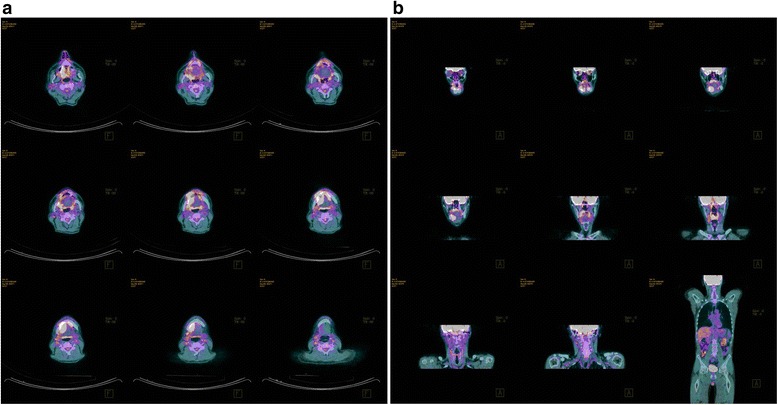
Preoperative ^18^F-FDG PET/CT. 2a axial plane; 2b coronal plane
